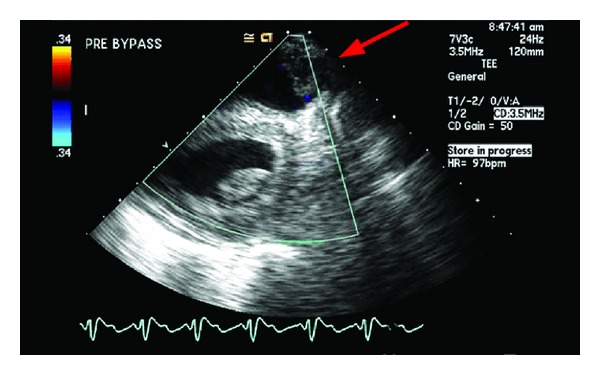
Intraoperative epicardial ultrasound imaging of the right ventricular outflow tract (RVOT) and pulmonary conduit. Arrow: filling defect obstructing the RVOT.
